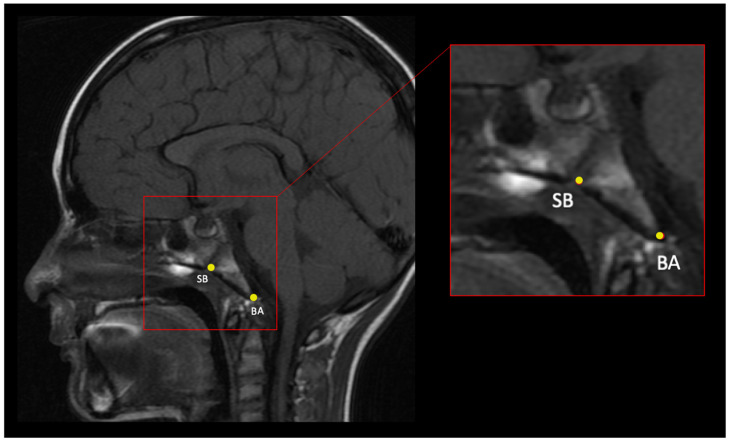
Midsagittal slice from an MRI scan depicting basion (BA) and sphenobasion (SB).
